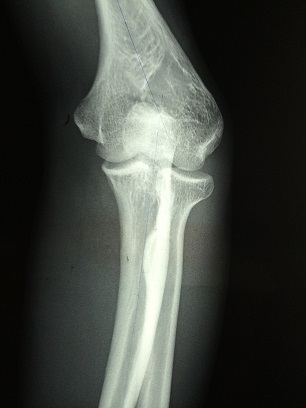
Radiographie de face montrant la deformation en varus
